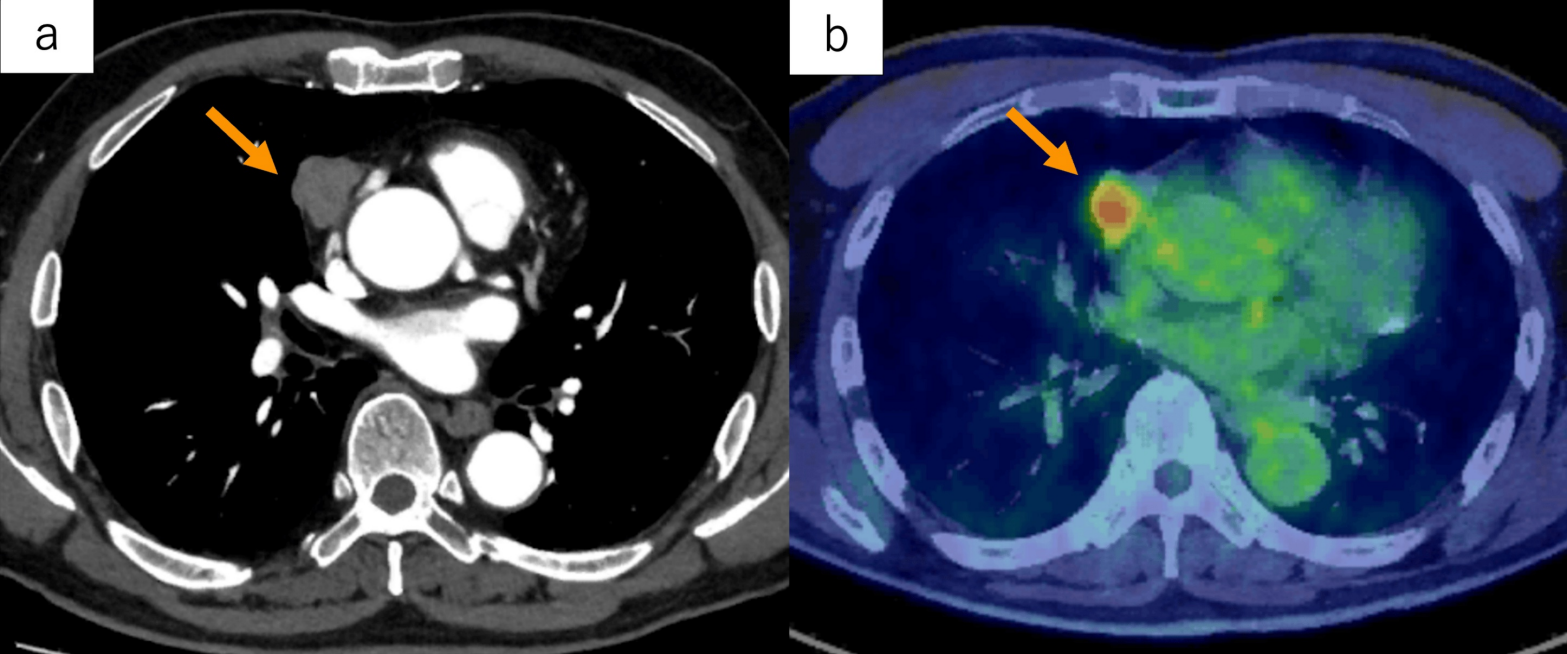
Imaging findings of the anterior mediastinal lesion. (a-b) Computed tomography scan and positron emission tomography-computed tomography scan showing a 27-mm tumor noted at the anterior mediastinum with fluorodeoxyglucose accumulation (SUVmax: 4.65) (orange arrows). SUVmax: maximum standardized uptake value.
